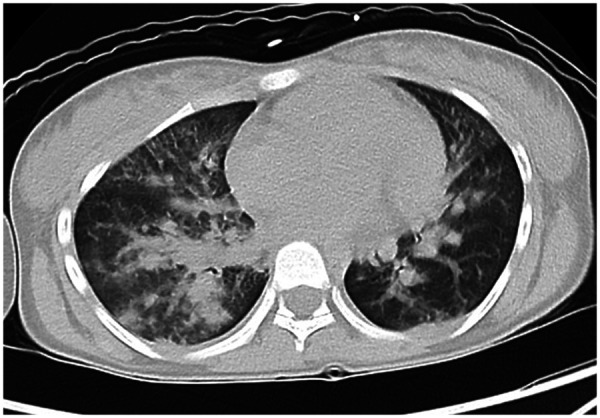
Chest CT showing bilateral multifocal pulmonary abnormalities, including patchy and nodular opacities, linear densities, and ground-glass opacities. Accompanying findings included thickening of the interlobular septa and interlobar pleura, as well as bilateral lower lobe pleural thickening.
